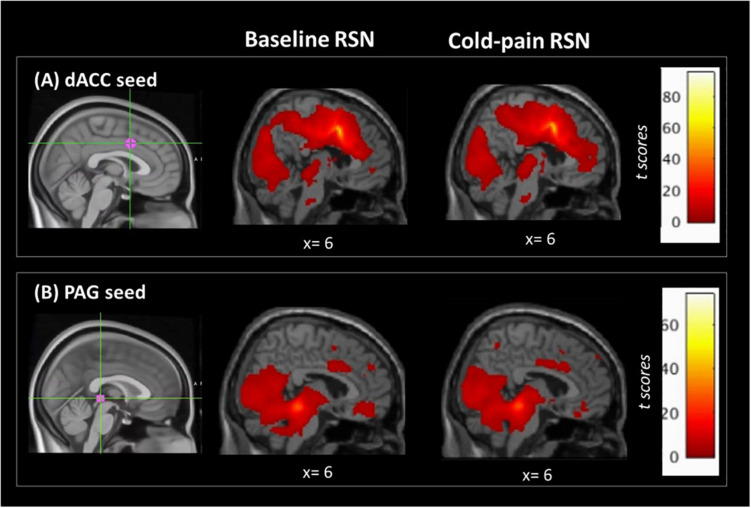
Regions of interest used in the seed-based analysis and their associated RSNs at baseline and during Cold-pain. (A) dACC ROI, MNI_xyz_ = (2, 8, 38). This ROI had a 7 mm spherical radius. Anatomically, the dACC-seed lies in Brodmann area 24. (B) PAG ROI, MNI_xyz_ = (0, –30, –1), the size was 3 mm spherical radius, and the seed was positioned in the anatomical midline. ROIs (in magenta) are overlaid on MNI-standardized T1-weighted images for visualization purposes. Color bars indicate *t* scores.
